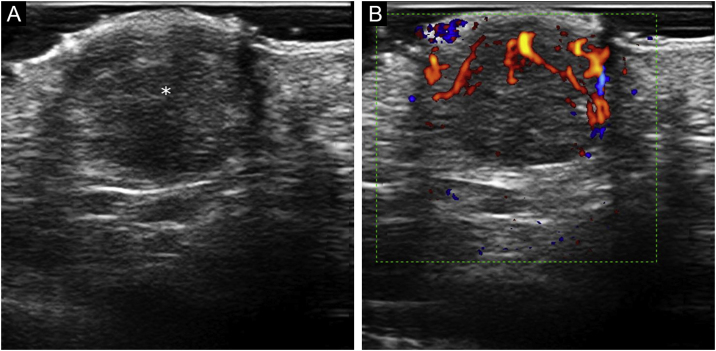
Sonographic characteristics of volar adult xanthogranuloma. (A) Grayscale sonogram (transverse view, right palm) showing a well-defined hypoechoic, dermal, and hypodermal nodule (asterisk) displacing the epidermal layer upwards; (B) Color Doppler sonogram of the same lesion showing inner vascularity.
